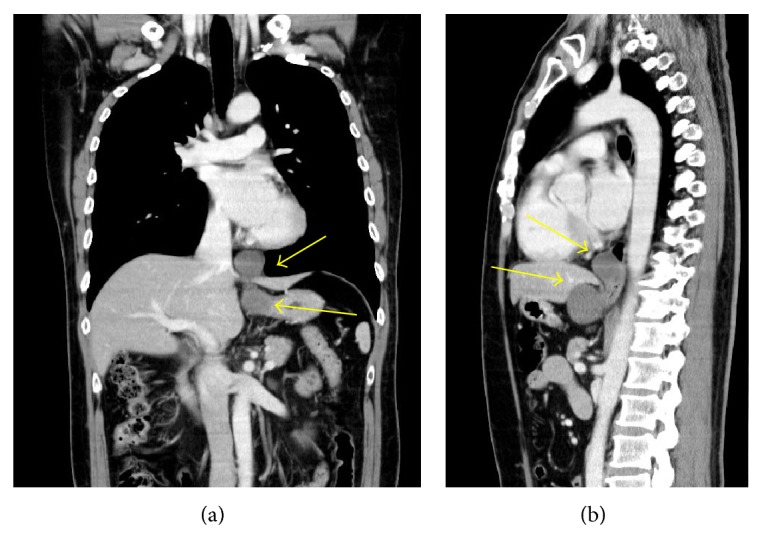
Computed tomography. The images reveal a dumbbell-shape iso-enhanced mass with a smooth surface, which extends parallel to the esophagus and spreads over and below the esophageal hiatus.
